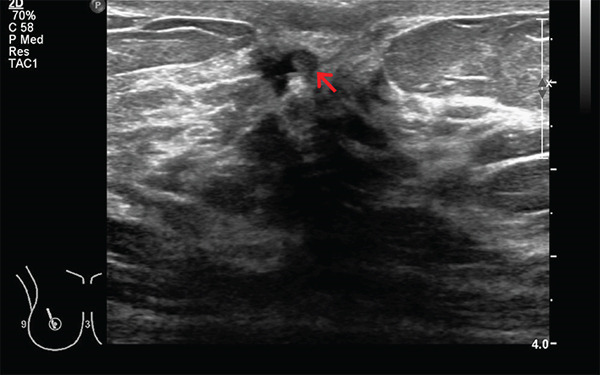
Ultrasound of the right subareolar breast in antiradial projection demonstrates a 3‐mm intraductal, solid mass at the 1 o′clock position (red arrow). BI‐RADS 4 (suspicious; biopsy recommended).
